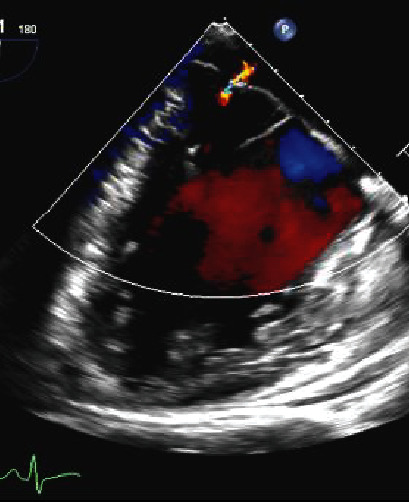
Transoesophageal systolic frame demonstrating MAD, as well as bileaflet MV prolapse and mild mitral regurgitation.
